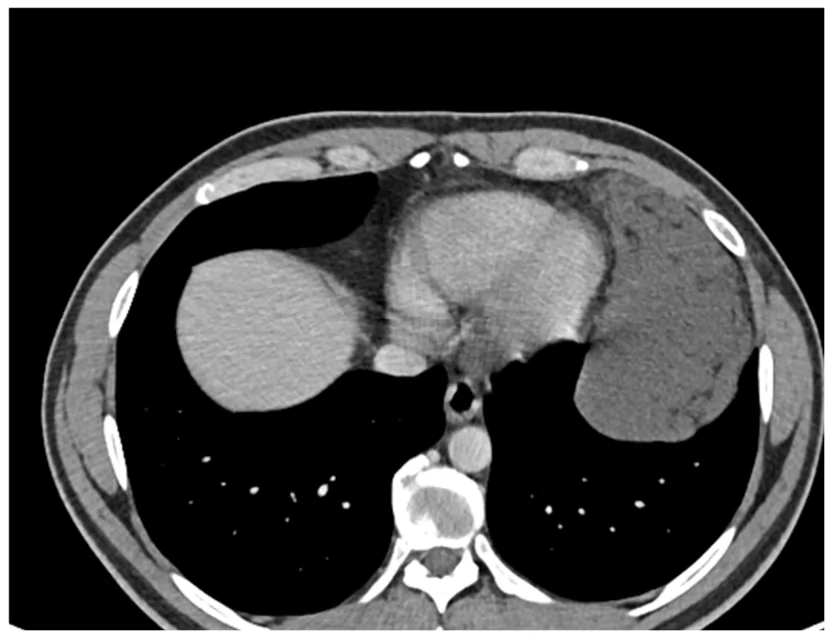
Axial CT scan displaying a heterogeneous 100×65 mm mass with fluid and adipose attenuation components in the left supradiaphragmatic costophrenic recess.
